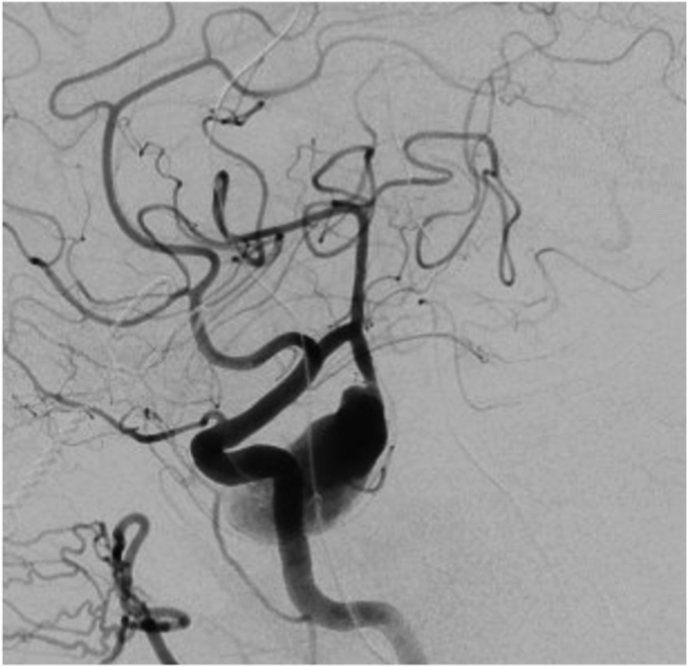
Diagnostic Images of Case #2.* A) Diagnostic MRI showing the partially thrombosed distal MCA aneurysm. B Digital Subtraction Angiography, left ICA injection, lateral view showing the large fusiform aneurysm.
